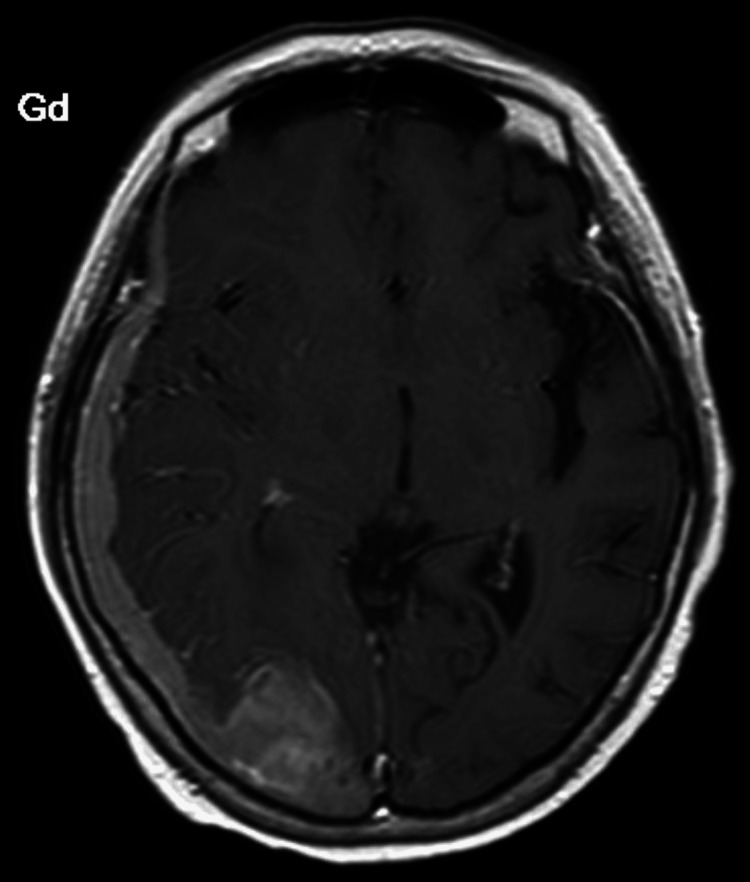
Contrast-enhanced brain MRI after chemotherapy showing enlargement of the mass with heterogenous contrast enhancement, and dural thickening worsened from the right temporal area to the parietal area
